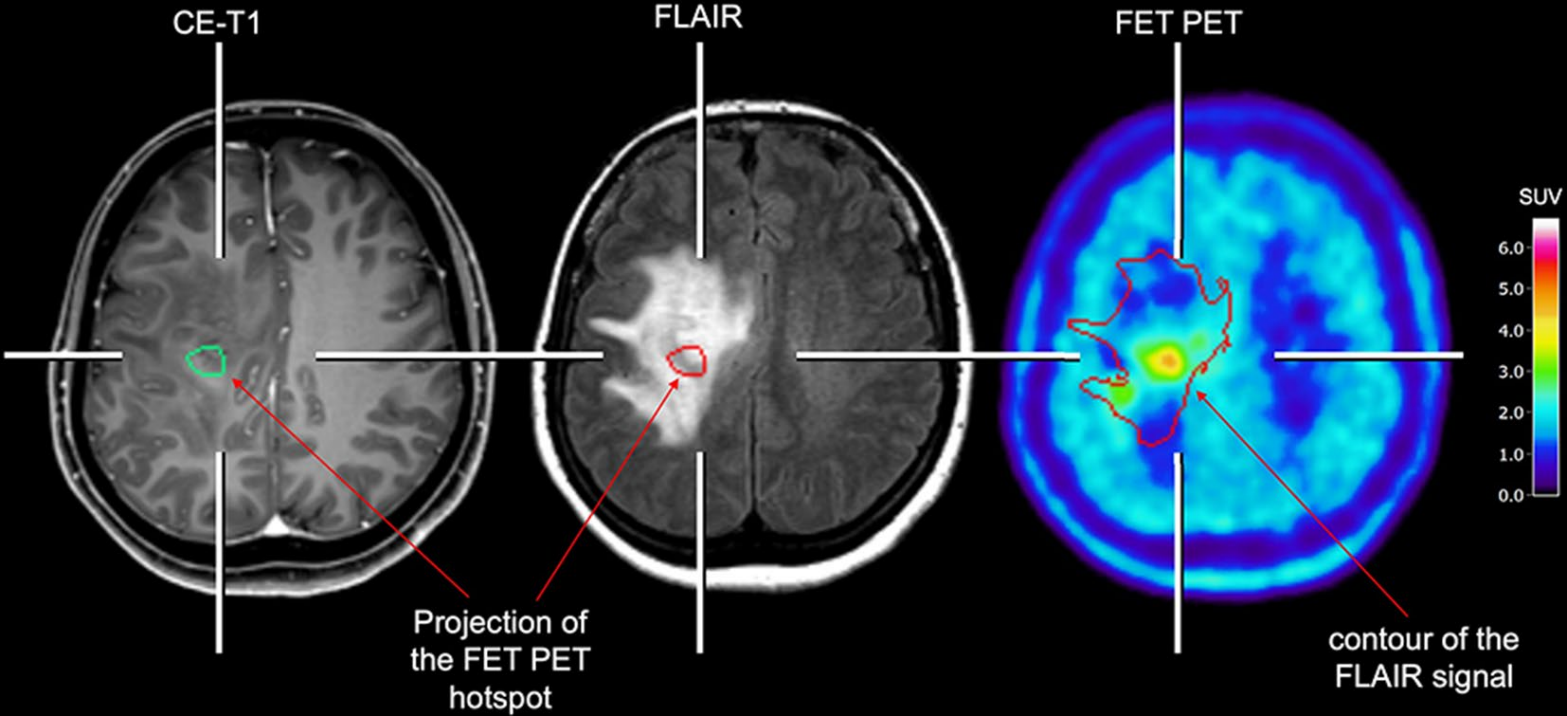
Contrast-enhanced MRI and FET PET of a 49-year-old female patient with a large FLAIR-hyperintense lesion without contrast enhancement in the right central cortex suggesting a glioma. Due to the widespread FLAIR signal, a definite biopsy target is lacking. The additional FET PET scan shows a localized area with pathologically increased metabolic activity (maximum tumor-to-brain ratio, 3.3), considerably smaller than the hyperintense FLAIR signal (red contour), and thereby offers a target for diagnostic biopsy. After FET PET-guided stereotactic biopsy of the metabolically active lesion, neuropathological evaluation of the obtained tissue revealed an IDH-mutant astrocytoma, WHO CNS grade 2
